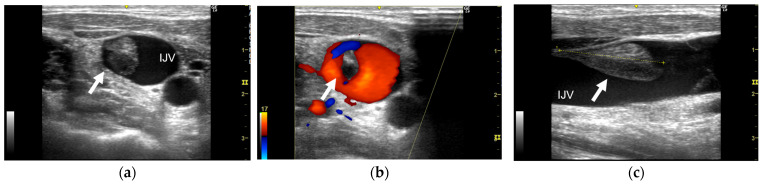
Ultrasonographic images of an IJV thrombus (arrows) confirmed after catheter removal. (a): transverse section; (b): color Doppler imaging; (c): longitudinal section. Color Doppler imaging shows blood flow outside the thrombus. IJV, internal jugular vein.
